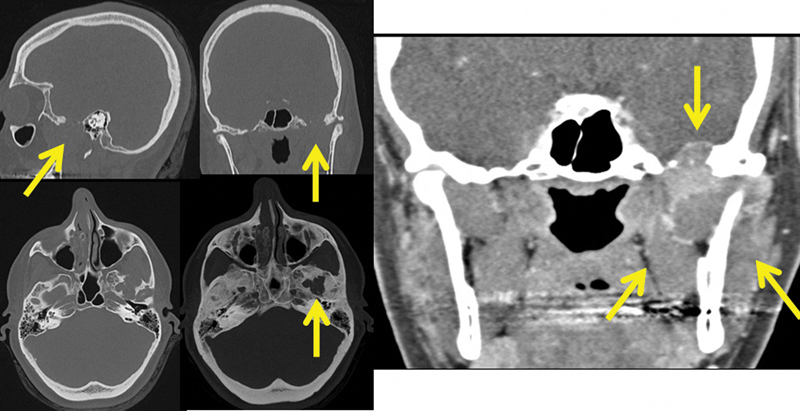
Computed tomography of the skull revealed an osteolytic lesion in left temporomandibular joint (arrows). Lesion had slight contrast enhancement.
